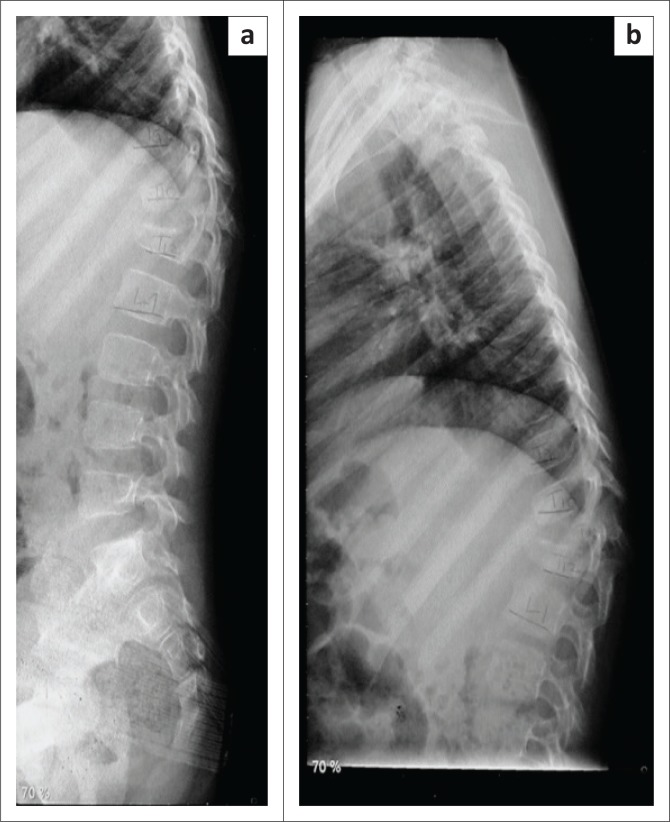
X-rays showed (a) L1 upper end plate and (b) T12 lower end plate destruction with peri-vertebral shadow.
